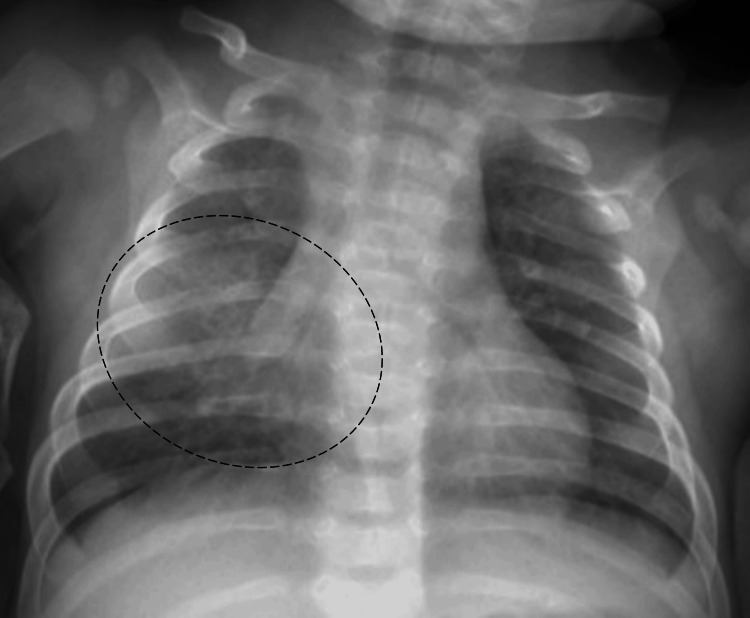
Chest radiograph at admission Anteroposterior chest radiograph showing a right opacity, resulting in an obliteration of the cardiac silhouette (circle).
